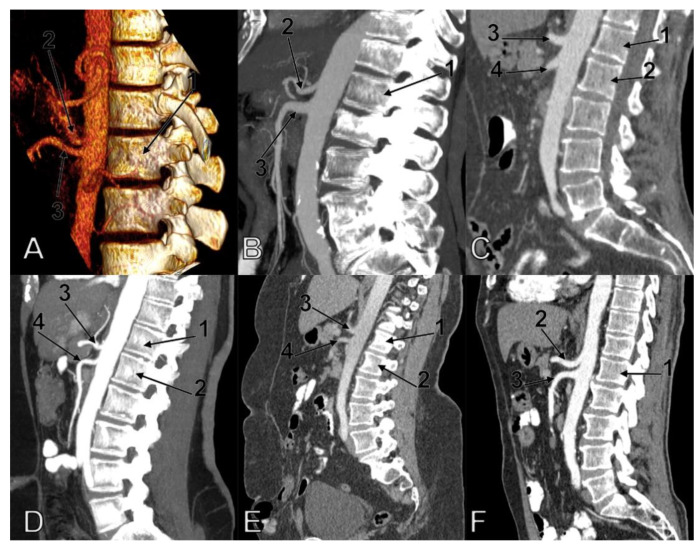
Combined patterns of the vertebral levels (types) of the aortic origins of the celiac trunk (CT) and superior mesenteric artery (SMA). (A) Three-dimensional volume rendering of a 5/6 type combination, left lateral view (1.1st lumbar vertebra; 2.CT; 3.SMA). (B) Sagittal slice of a 6/9 type combination (1.1st lumbar vertebra; 2.CT; 3.SMA). (C) Sagittal slice of a 7/9 type combination (1.1st lumbar vertebra; 2nd lumbar vertebra; 3.CT; 4.SMA). (D) Sagittal slice of a 4/6 type combination (1.12th thoracic vertebra; 2.1st lumbar vertebra; 3.CT; 4.SMA). (E) Sagittal slice of a 6/8 types combination (1.1st lumbar vertebra; 2.2nd lumbar vertebra; 3.CT; 4.SMA). (F) Sagittal slice of a 5/7 types combination (1.1st lumbar vertebra; 2.CT; 3.SMA).
